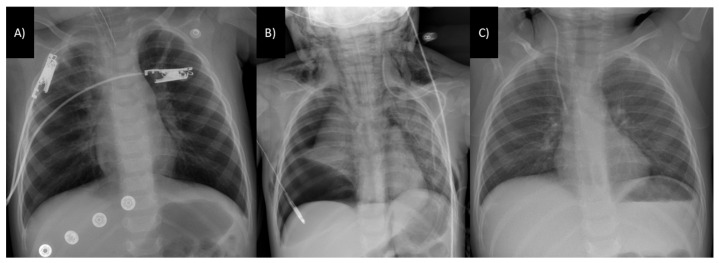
Chest radiographs: (A) upon admission, a chest radiograph depicted bilateral perihilar alveolar infiltration; (B) chest radiograph performed on hospital day seven showed subcutaneous emphysema, pneumomediastinum, and massive right pneumothorax; (C) at discharge, the chest radiograph displayed a normal aspect.
